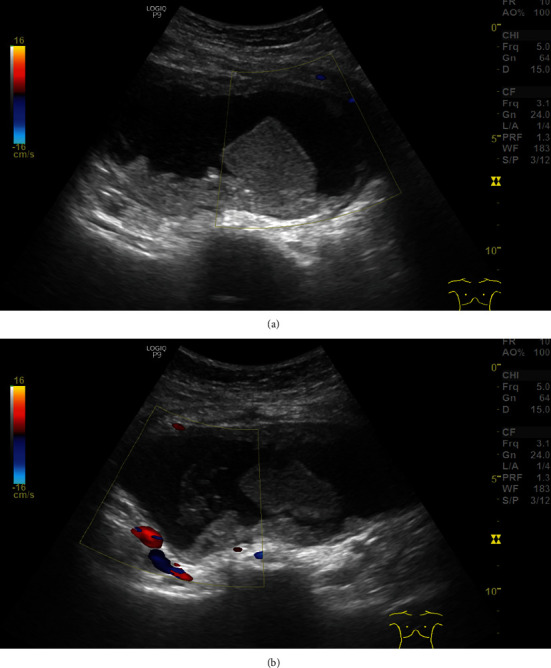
Ultrasound images of persistent left ovarian cyst (3 months later). A hypoechoic cyst with a few polygonal shape density lesions inside the ovarian area with the hypogastric vessel as a landmark was shown on the images. (a) A hypoechoic cyst with a few polygonal shape lesion inside. (b) The hypogastric vessel as a landmark of the ovary.
